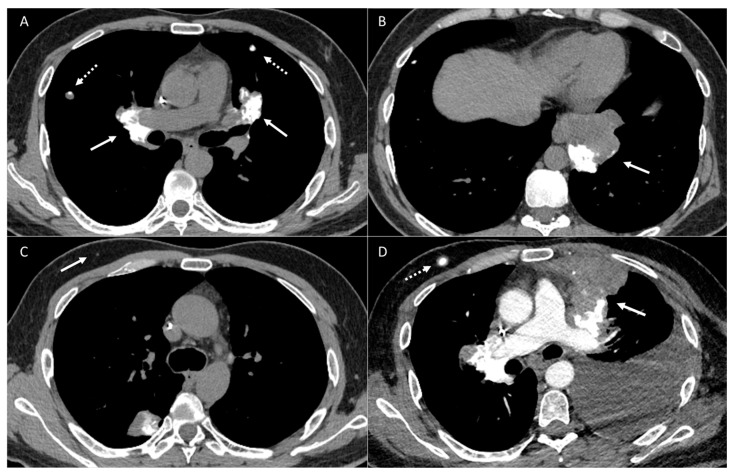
Patient with osteosarcoma multi-compartment thoracic metastasis. Axial non-enhanced image (A) shows bilateral calcified/ossified metastatic pulmonary nodules (arrows) and hilar lymphadenopathy (dashed arrows). Axial non-enhanced image (B) shows a left partially calcified/ossified pleural-based metastatic mass (arrow). Axial non-enhanced image (C) shows a small right anterior chest wall subcutaneous nodule (arrow) and a right lung subpleural nodule with calcification. Axial enhanced image (D) from a follow-up CT shows an interval increase in the size of the metastatic subcutaneous nodule with new calcification/ossification (dashed arrow), development of a left anterior pleural-based metastatic mass (arrow), and new left large left pleural effusion.
